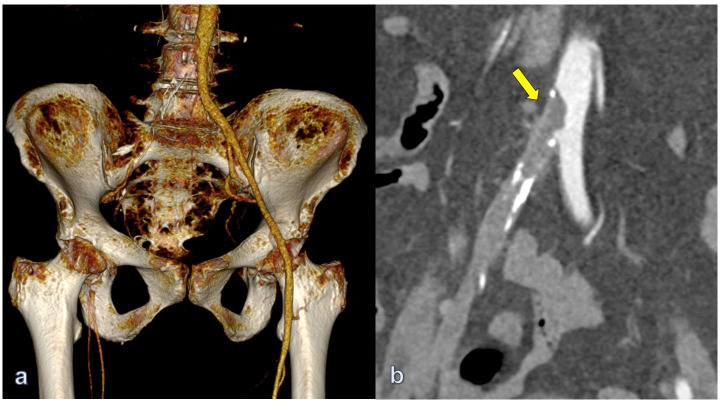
Preoperative computed tomography angiography (angio CT) with volume rendering 3D reconstruction: thrombotic occlusion of the right common and external iliac arteries with patent right femoral bifurcation. Of note, healthy and patent left Ilio-femoral axis (a). Iliac intraluminal thrombus protruding into aorta (arrow) (b) (patient #1).
